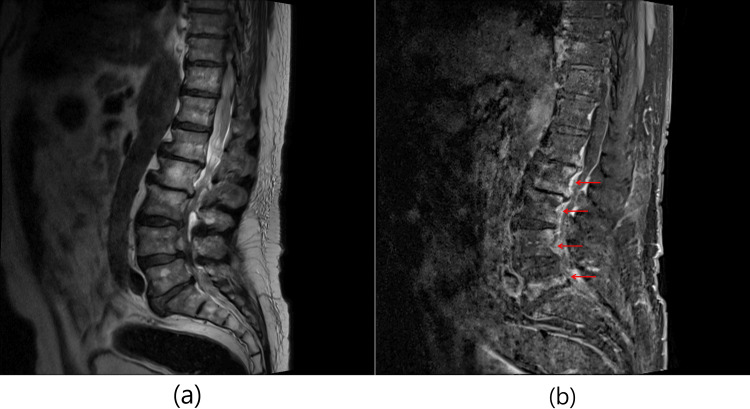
Spine sagittal magnetic resonance imaging (MRI) was obtained at diagnosis. (a) T2-weighted image demonstrates endplate destruction and decreases height of disc space of L5/S1 and thickening along the epidural space below L1 level. (b) Contrast-enhanced T1-weighted image with fat suppression demonstrates subtle bone marrow enhancement at the posterior body adjacent to the spinal canal of L2, L3, and L4 and enhancement along the epidural space below the L1 level (red arrow).
